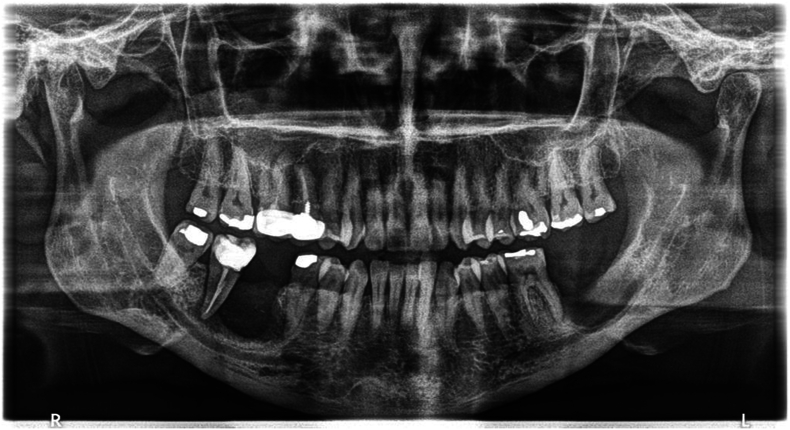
Panoramic x-ray assessment before surgery.
